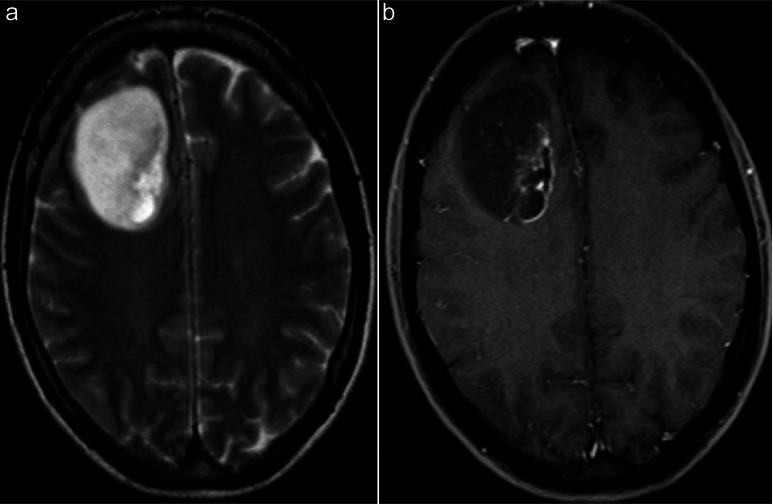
The primary tumor is well circumscribed with patchy contrast enhancement in the right frontal lobe. T2-weighted (a) and post-contrast T1-weighted (b) images.
